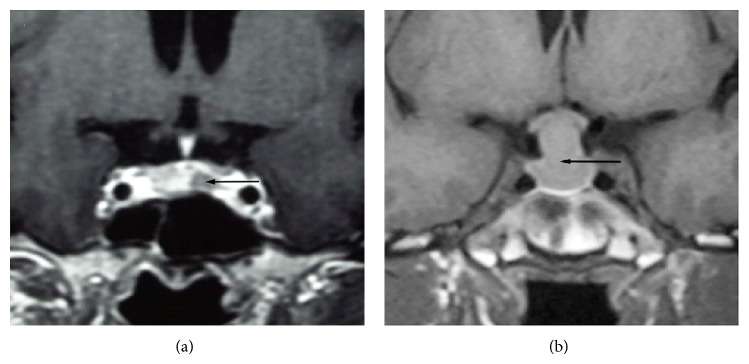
Representative T1-weighted coronal MRI scans. (a) Microadenoma (arrow). (b) Macroadenoma expanding into the suprasellar cistern (arrow).
